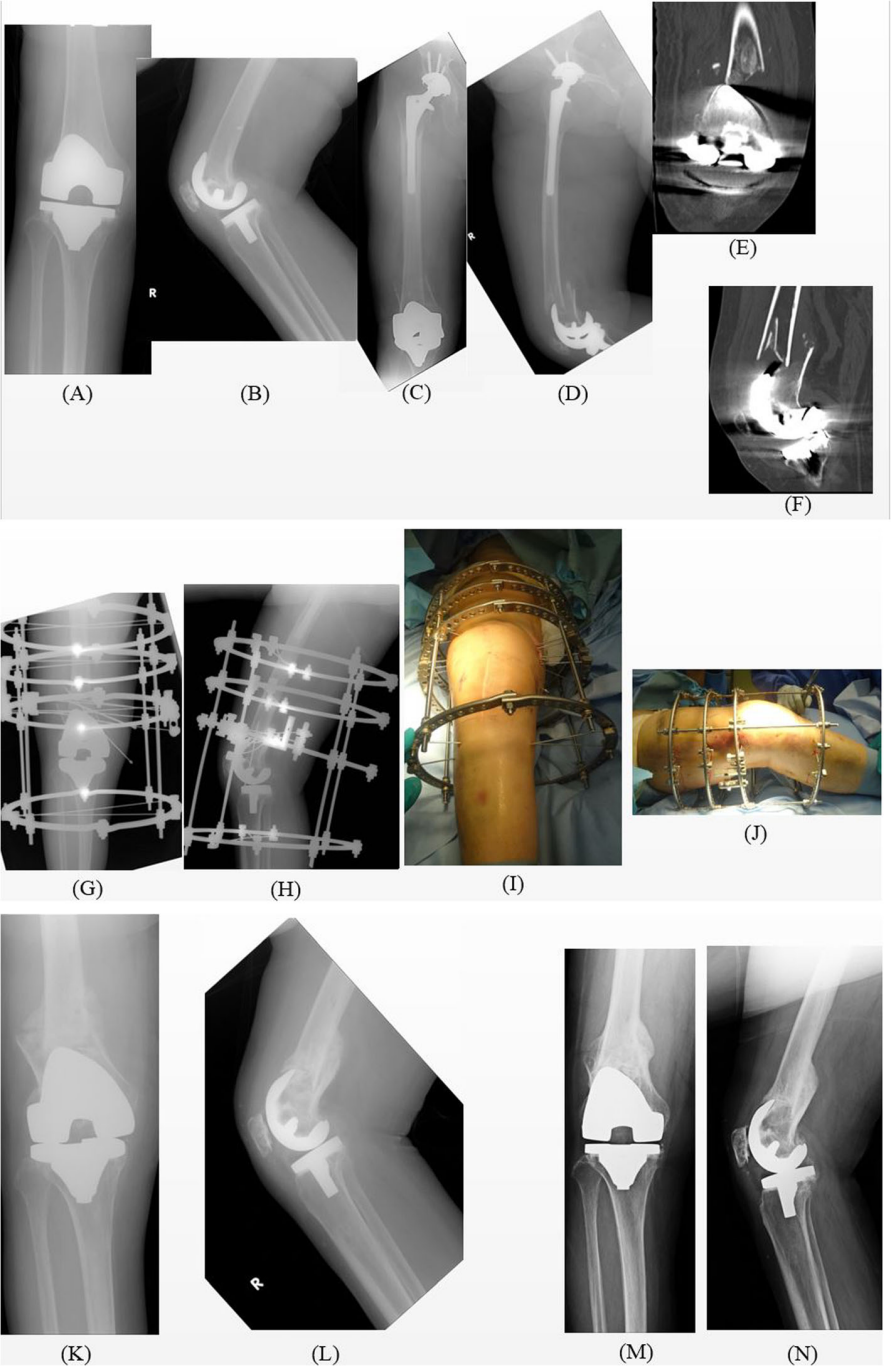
Periprosthetic distal femur fracture in case number 5 treated with a circular external fixator. (A, B) The joint before injury. (C) Preoperative antero-posterior X-ray after the injury. (D) Preoperative lateral angle X-ray. (E) Preoperative coronal CT scan. (F) Preoperative sagittal CT scan. (G, H) Immediate postoperative X-ray. (I, J) Immediate postoperative clinical photograph. (K, L) X-ray after removal of the circular external fixator and weight-bearing (10 weeks after the surgery). (M, N) Three years after the surgery. CT, computed tomography
